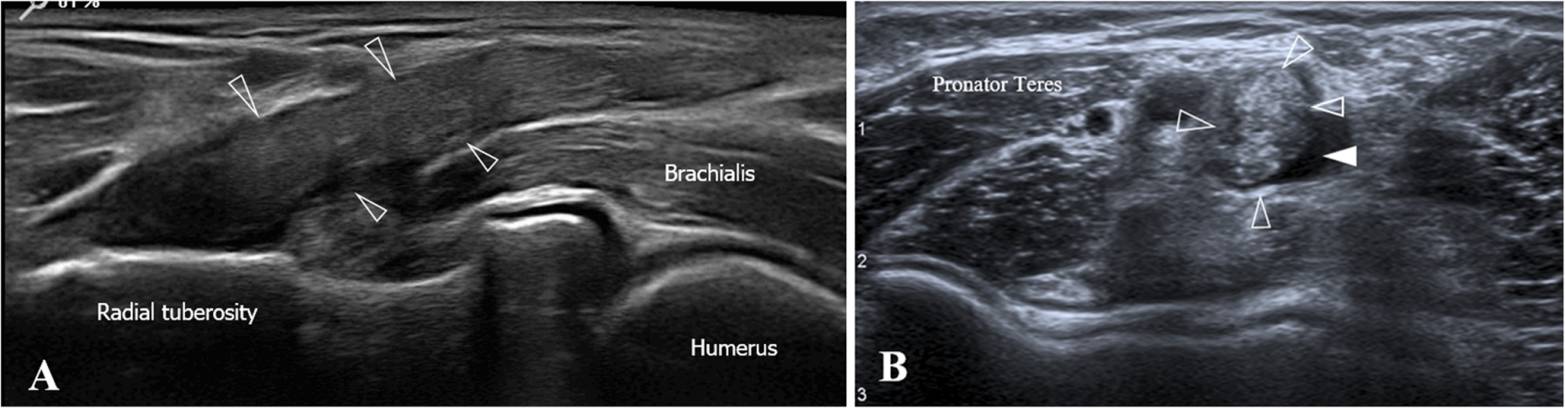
Ultrasound view of a type 1B injury (elongation or traumatic injury without evidence of tendon tearing). a In the long-axis view, the DBBT (*empty arrowheads*) is uniformly thickened and hypoechogenic, with a loss of the fibrillary pattern affecting its whole thickness and length. However, there are no signs of tendon tearing. b Short-axis view of a. The DBBT cross-section shows that the alteration affects both tendon components (*empty arrowheads*), which are observed as being thicker and having an altered echostructural pattern. Also, a localised peritendinous effusion is observed (*white arrowhead*)
